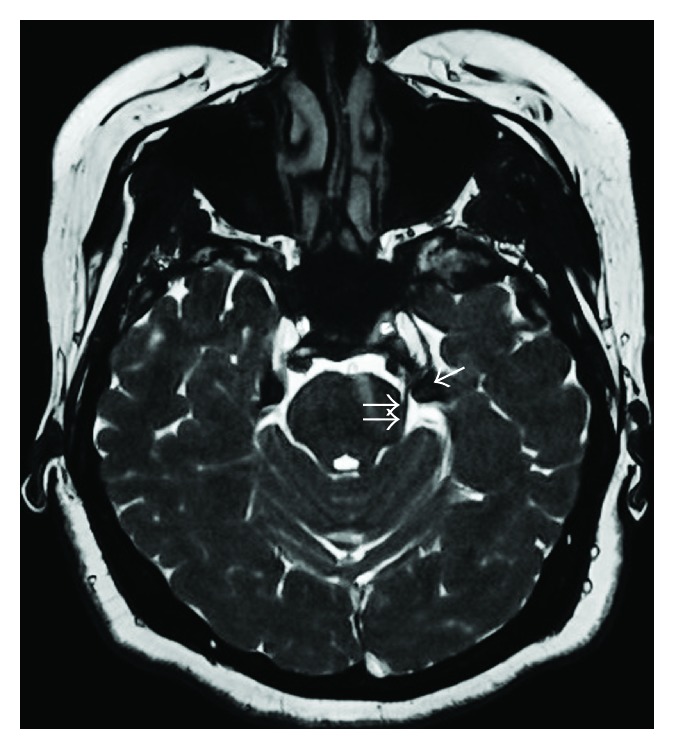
Axial 3D FIESTA MR image shows nodular lesions (arrow) with dark signal intensity adjacent to the porus trigeminus bilaterally. The cisternal segment of the left trigeminal nerve (double arrows) is impinged by nodular lesions.
